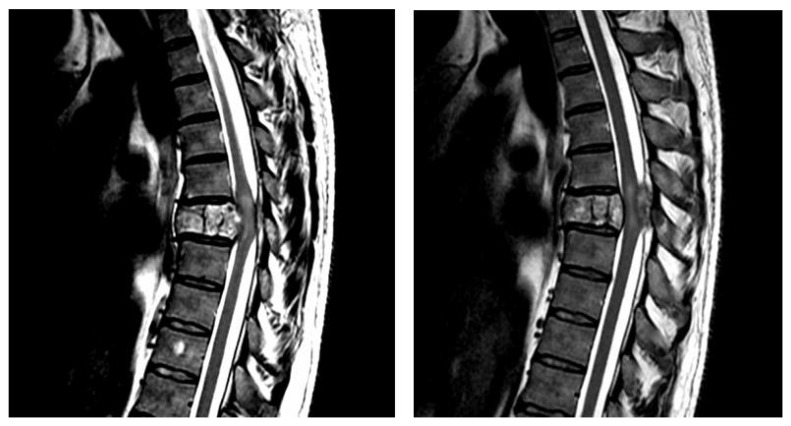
Spinal Cord Injury visualized on MRI-T2 sequence.
